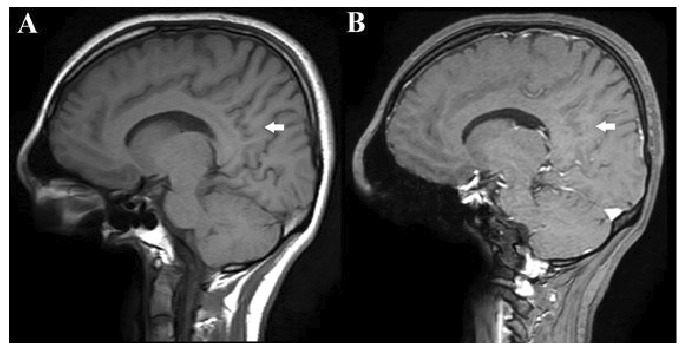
Follow-up sagittal T1-weighted images obtained after 1 month of antimicrobial therapy. The precontrast image (A): shows no visible lesion, and the post-contrast image (B): demonstrates no enhancement at the site of the previously observed lesion (arrow), indicating resolution of contrast uptake and radiological improvement.
